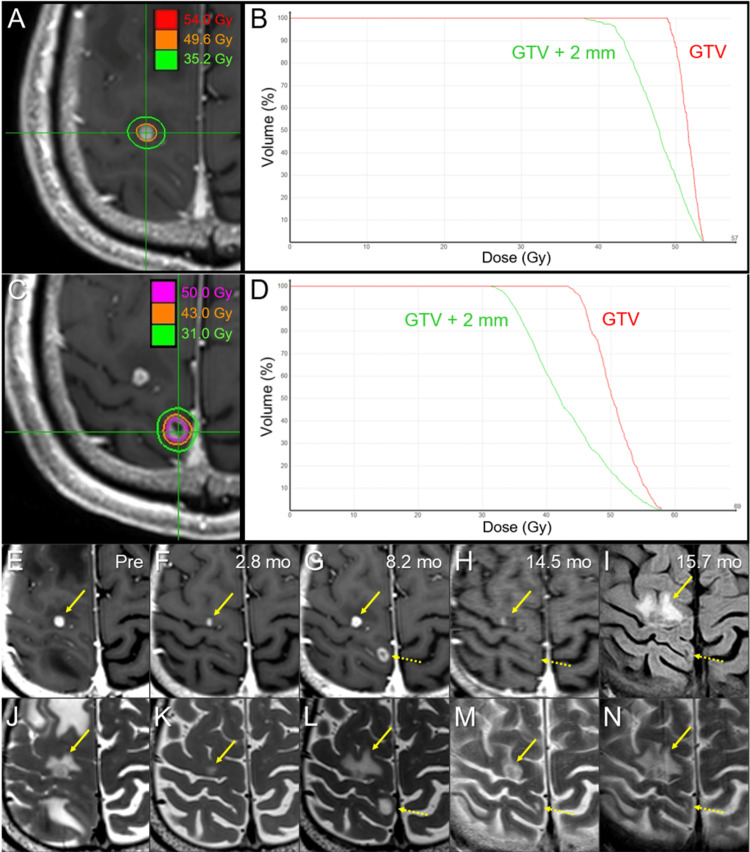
Dose distributions, dose-volume histograms, and magnetic resonance images before and after 8- and 5-fr stereotactic radiosurgery for the right frontal and parietal lesions, respectively. The images show dose distributions (A, C); DVHs (B, D); axial CE-T1-WIs (E-H); axial FLAIR image (I); axial T2-WIs (J-N); the right frontal lesion (A, B); the right parietal lesion (C, D); five days before the 8-fr SRS (E, J); at 2.8 months (mo) after the 8-fr SRS (F, K); at 8.2 months (before the 5-fr SRS) (E, J); at 14.5 months (6.1 months after the 5-fr SRS) (F, K); and at 15.7 (7.3) months (G, L). (C-L) These images are shown at the same magnification and coordinates under co-registration and fusion. (E, J) The solid enhancing lesion (arrow in E) associated with perilesional edema (arrow in J) in the right frontal lobe. (F, K) At 2.8 months after the 8-fr SRS, the lesion shrank obviously (arrow in F), and the perilesional edema almost disappeared (arrow in K). (G, L) At 8.2 months, the lesion obviously enlarged (arrow in G), and the perilesional edema expanded (arrow in L) more extensively before SRS. The new lesion (dashed arrows in G, L) appeared in the right parietal lobe. (H, M) The enhancing effect of the frontal lesion was attenuated (arrow in H), and the perilesional edema decreased (arrow in M). However, the visible mass on T2-WI enlarged (arrow in M), suggesting pseudo-response to chemoimmunotherapy. The parietal lesion shrank markedly, leaving only the cavitary scar (dashed arrows in H, M). (I, N) In the frontal lesion, the visible mass persisted, and the perilesional edema further expanded (arrows in I, N). The parietal lesion remained in nearly complete remission (dashed arrows in I, N). DVHs: dose-volume histograms; CE: contrast-enhanced; WI: weighted image; FLAIR: fluid-attenuated inversion recovery; fr: fraction; SRS: stereotactic radiosurgery; GTV: gross tumor volume
